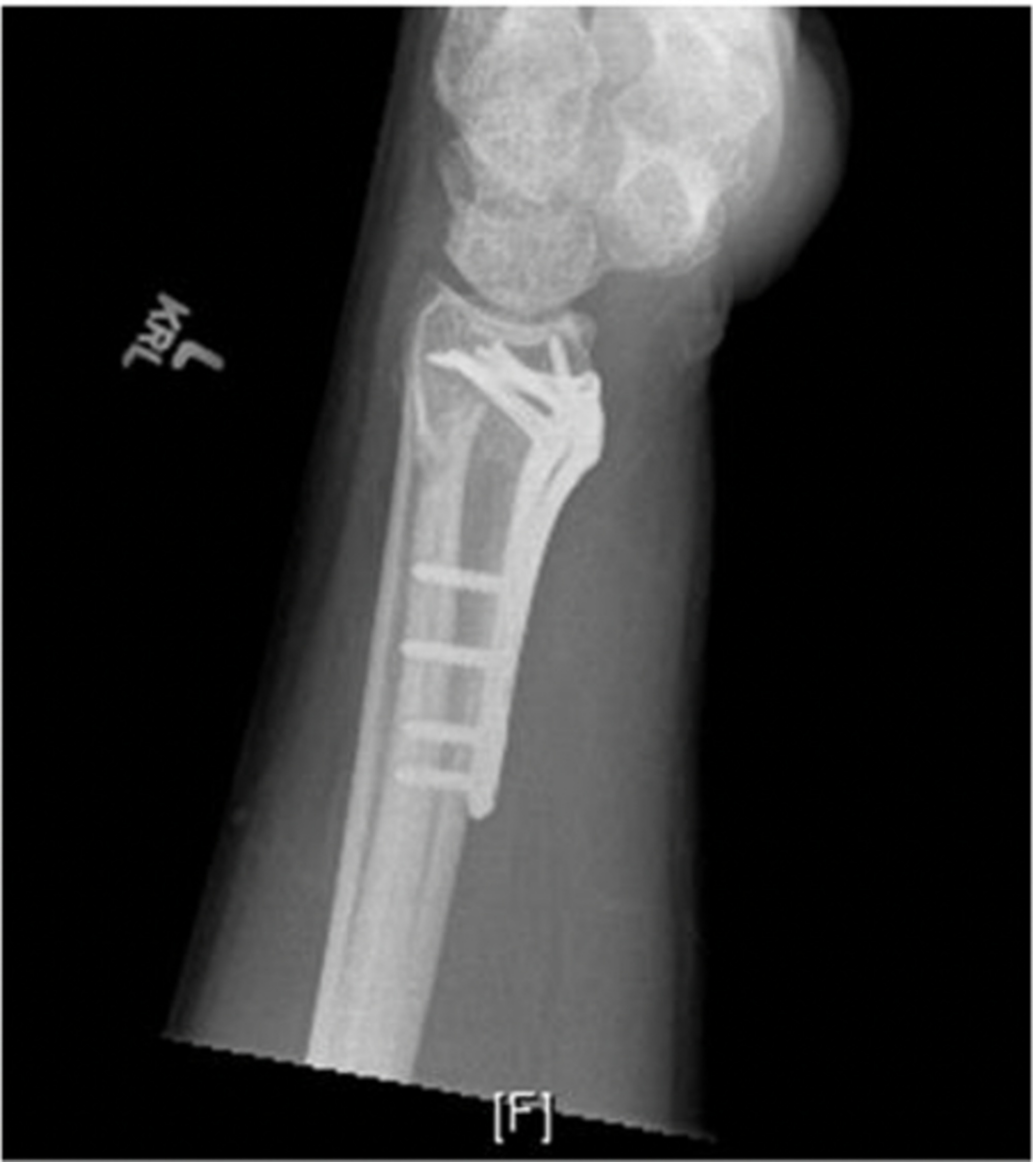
Lateral X-ray of the left wrist demonstrating volar plate fixation with a healed distal radius fracture and small displaced ulnar styloid fracture with appears stable from previous X-rays
